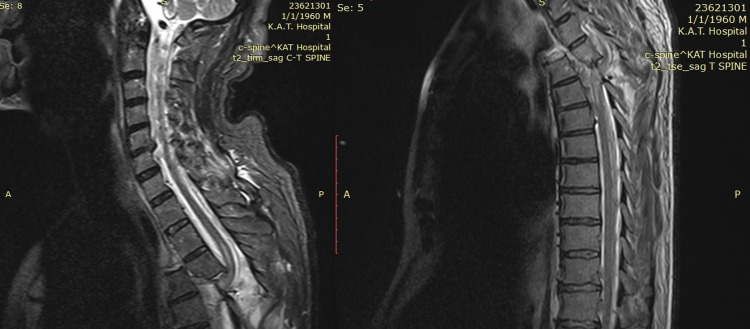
Thoracic MRI of the spine Sagittal T2-weighted MRI (right) demonstrating severe canal stenosis and cord compression at the level of dislocation, and STIR sequence (left) revealing disruption of all posterior ligaments. Intramedullary hyperintensity spanning T3-T4 present in both images likely reflects cord contusion.
